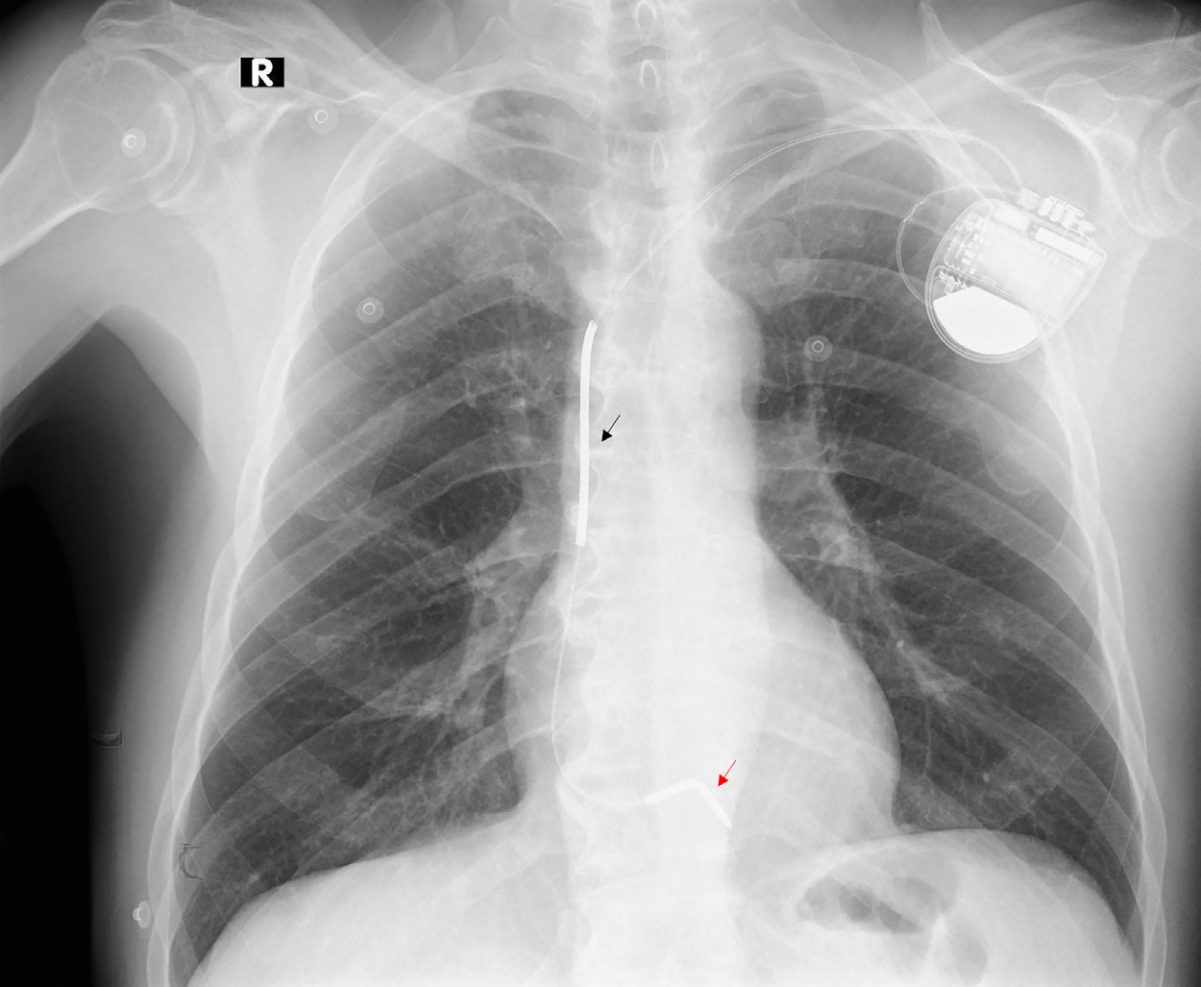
Chest X-ray after ICD placement. Frontal view chest radiography showing a single-chamber pacemaker with its characteristic shock coils in superior vena cava (black arrow) and another that surrounds RV lead (red arrow) in a 68-year-old man with biventricular arrhythmogenic cardiomyopathy with predominant LV involvement.
